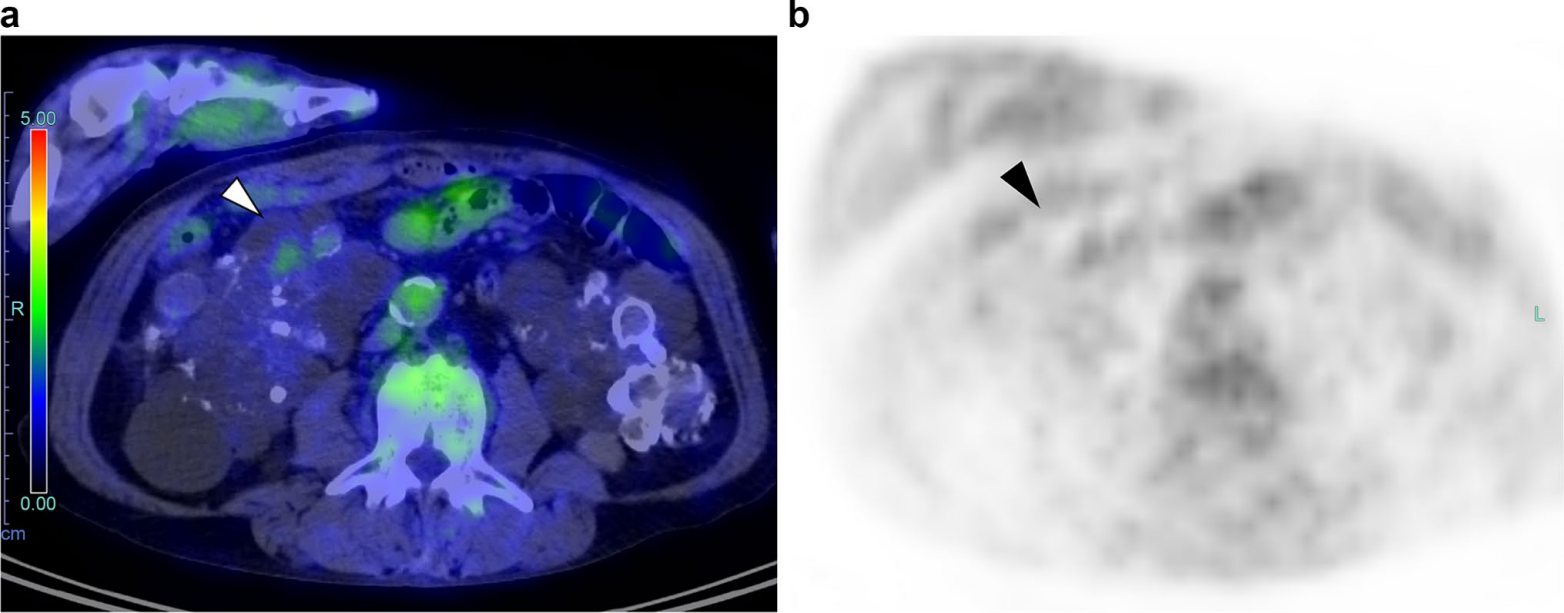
False-positive examination of a 62-year-old man FDG-PET/CT axial (a) and FDG-PET axial (b) images show FDG uptake with SUV_max_ of 2.0 in the right kidney (arrows). Additional examinations (non-contrast-enhanced MRI and ultrasonography) were performed; however, no tumor was identified. As of 4 years and 11 months of observation, no tumor has been identified. The cause of kidney disease was ADPKD
